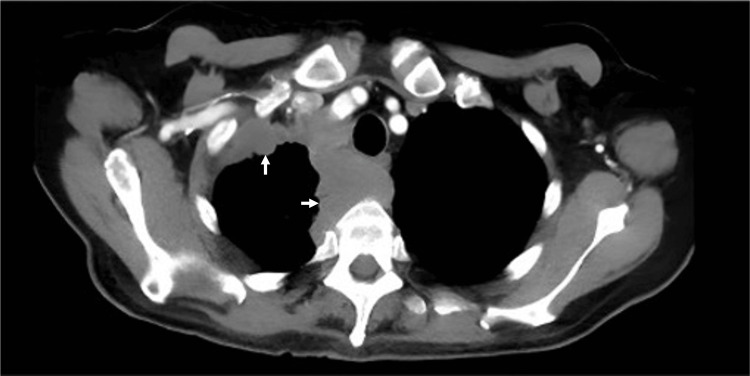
Computed tomography of the thorax (CT-thorax) demonstrating an irregular right apical pleural mass (white arrows) measuring 7.0 × 4.3 × 7.5 cm maximally, extending from the anterior to posterior mediastinum
